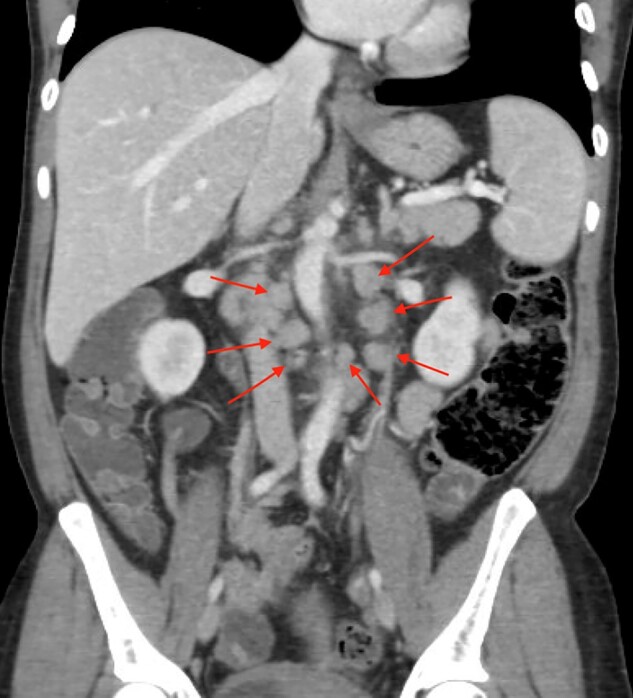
CT of the abdomen and pelvis showing lymphadenopathy (indicated by red arrows) in the retroperitoneum, with left para-aortic nodes as large as 2 cm and with multiple nodes at the base of the small bowel mesentery and multiple right and left para-aortic nodes
